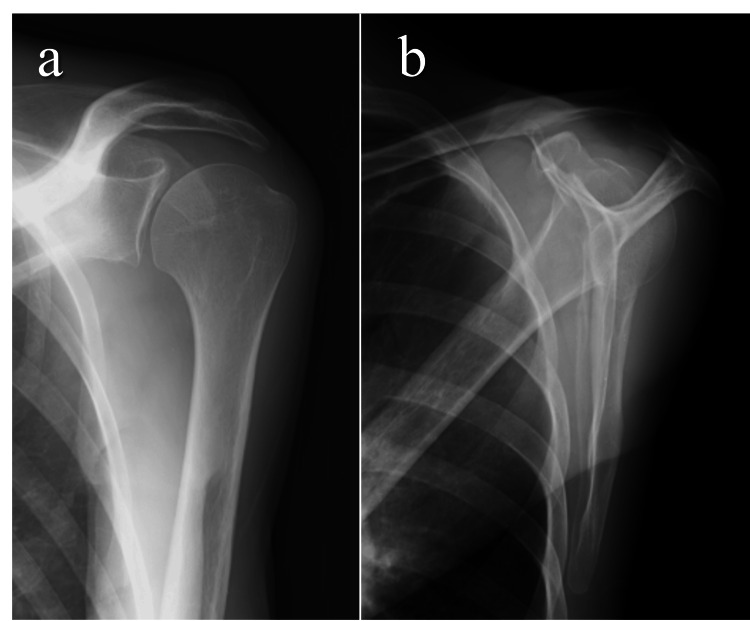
Plain radiographs obtained six years before referral to our institution. (a) Anteroposterior view and (b) scapular Y view showing no apparent osseous abnormalities or joint incongruity.
